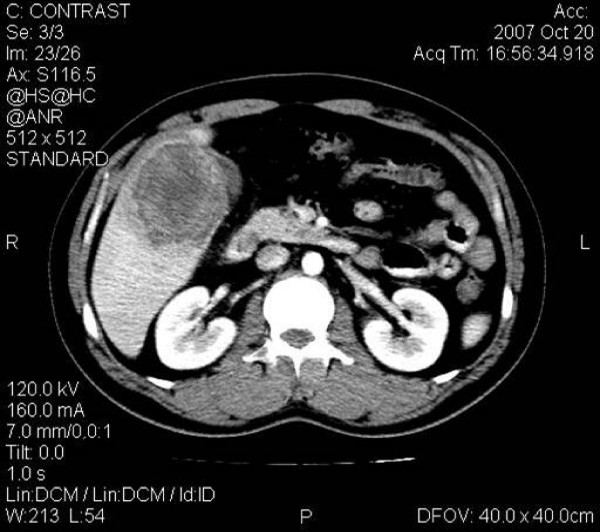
Computed tomography image. Computed tomography contrast-enhanced image, demonstrates a hemorrhagic lesion in liver segment V, presenting mild heterogeneous peripheral contrast enhancement.
